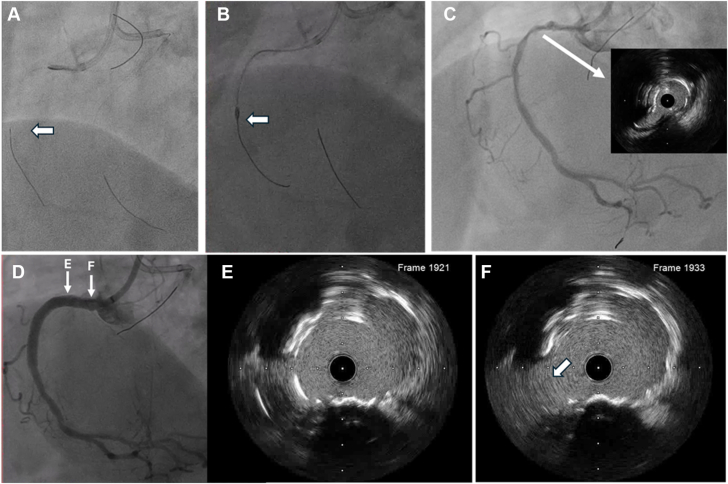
Rotational artherectomy and RCA CTO recanalization. (A-C) The antegrade RotaWire Extra Support guide wire successfully crossed the chronic total occlusion (CTO), enabling subsequent rotational atherectomy. Intravascular ultrasound confirmed a calcium fracture at the proximal CTO cap. (D-F) Following balloon angioplasty and a 3.5 × 48 mm stent deployment, the CTO was successfully recanalized. The arrow E highlights the stratified layer of the proximal CTO cap, while the arrow F indicates the plane of the sinoatrial nodal branch.
